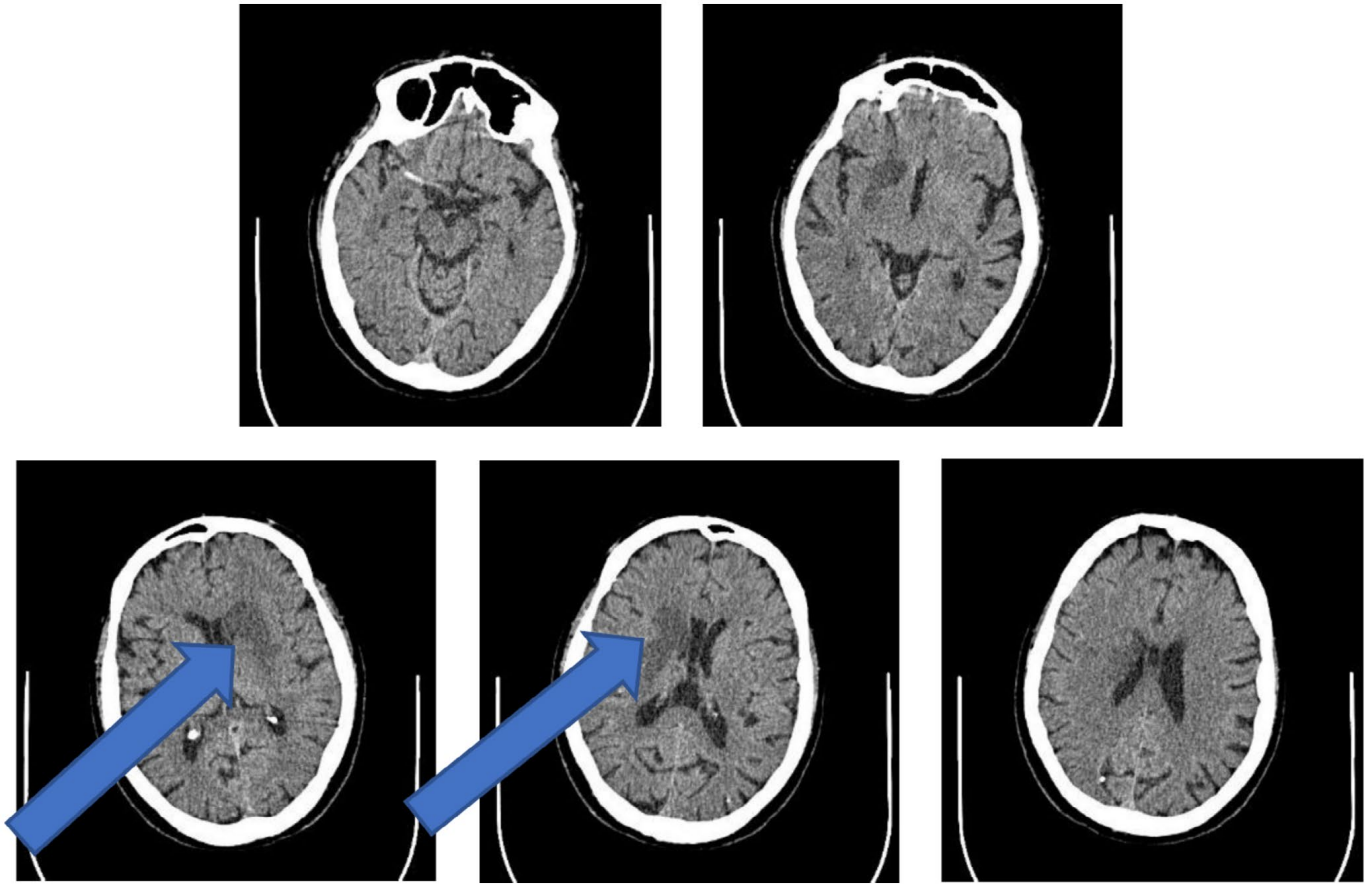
CT scan of the brain showed a 4.6 cm deep parenchymal hypodensity in the region of the right basal ganglia, suggestive of a subacute ischemic infarct (pointed by the arrow). No intracranial bleed, midline shift, hydrocephalus or masses were noted. Those findings in the clinical context were suggestive of an embolic stroke.
